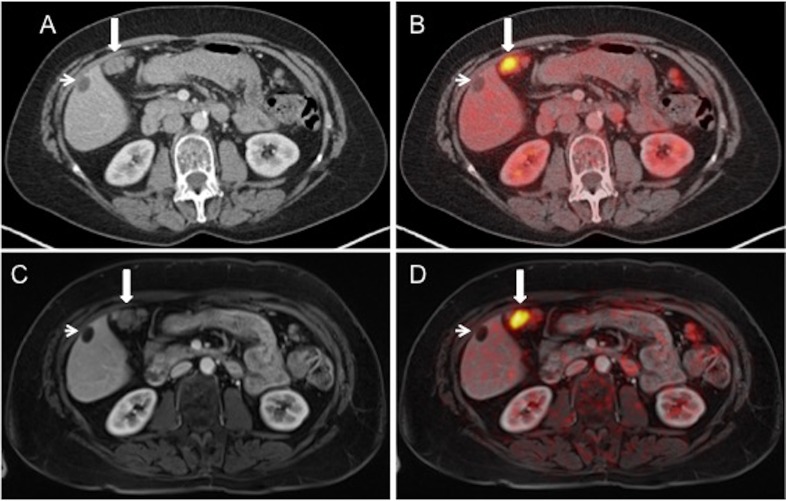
Images of a 58-year-old patient with recurrence of ovarian cancer. CT image (A) shows a peritoneal mass next to the liver with pathological FDG metabolism in fused PET/CT images (B). The same lesion is visible in post-contrast media VIBE sequence in MRI (C) and in PET/MRI (D). No FDG metabolism can be seen in a benign liver cyst in PET/CT or PET/MRI (A-D).
